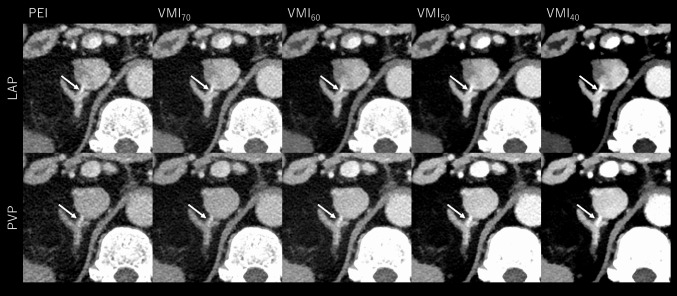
CT images of a 65-year-old male with PA (BMI: 29.1 kg/m^2^). The upper and lower rows display late arterial phase (LAP) and portal venous phase (PVP) images, respectively, reconstructed with polyenergetic images (PEI) and virtual monoenergetic images at 40–70 keV (VMI_40-70_). The window display is optimized for appropriate RAV assessment for each image series. In VMI, the contrast and depiction of the right adrenal vein (RAV, arrows) directly draining into inferior vena cava were maximized at VMI_40_. The perceived image noise in VMIs decreases as energy levels decrease, as a wider window display was used for lower-energy images to optimize image interpretation
